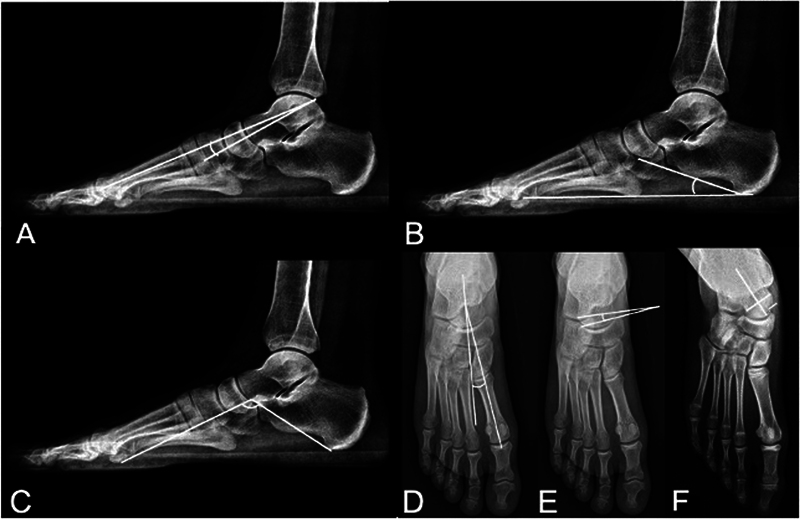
X-Ray evaluation of the foot deformities. (
